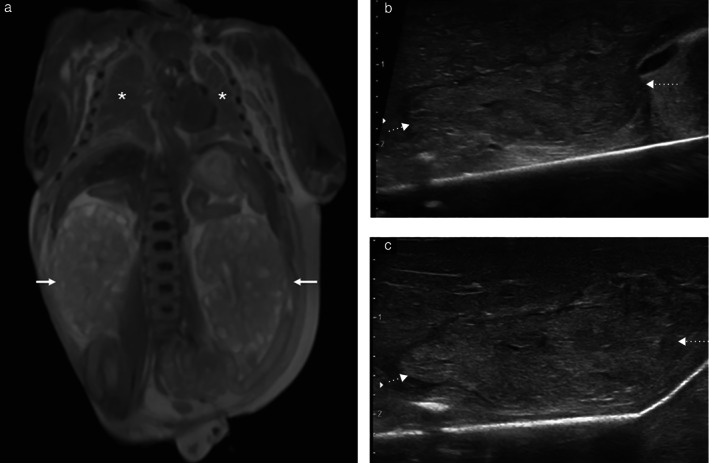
Postmortem magnetic resonance imaging and ultrasound images in 20‐week fetus. (a) Coronal T2‐weighted image of thorax and abdomen demonstrating enlarged cystic kidneys (arrows) with small hypoplastic lungs (
